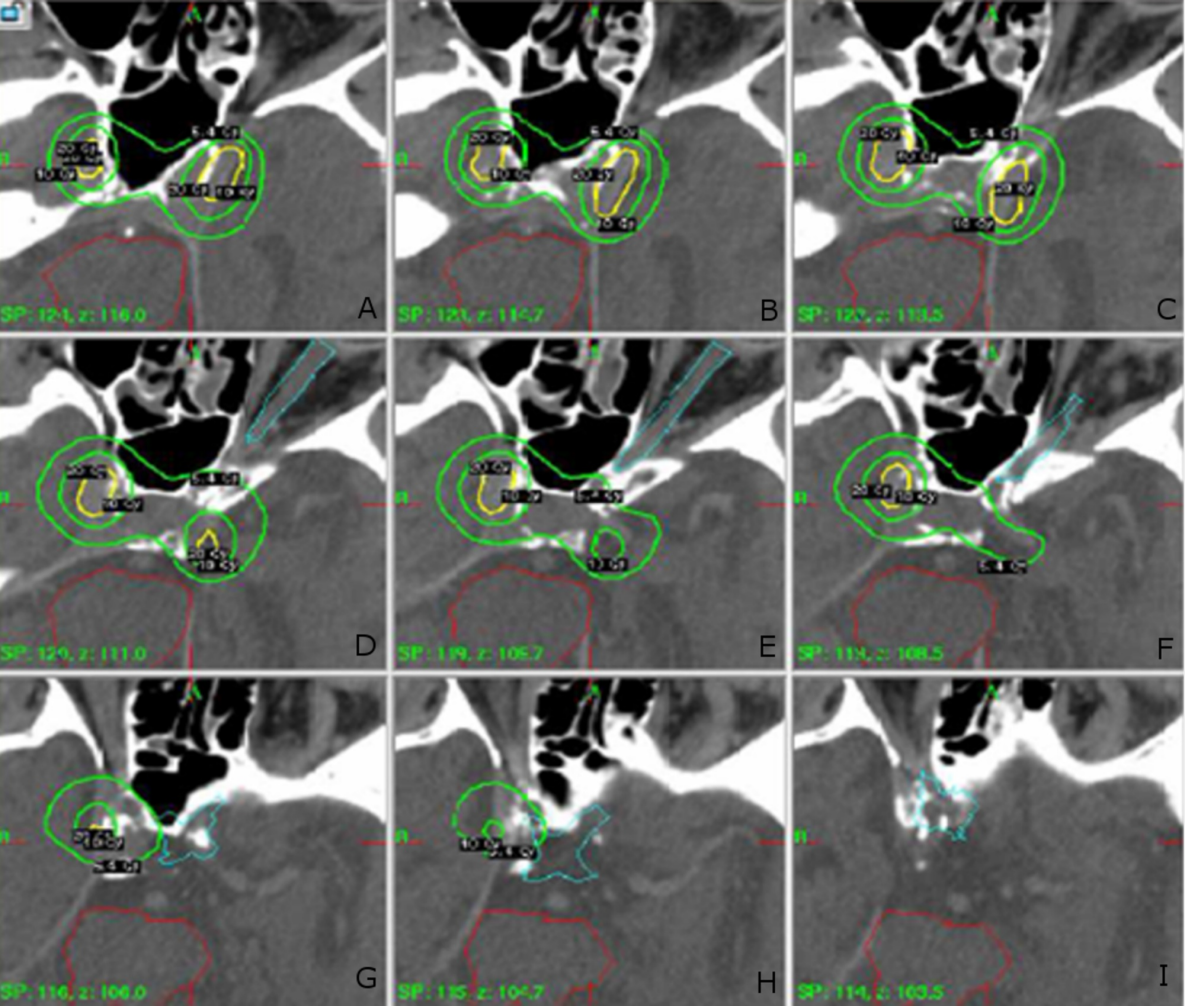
Gamma Knife Treatment Plan (A-I) CT scan with Gamma Knife treatment plan overlay. Outer green contour, 5.4 Gy. Inner green contour, 10 Gy. Yellow contour, 20 Gy. Turquoise contour, optic apparatus. Red contour, brainstem.
